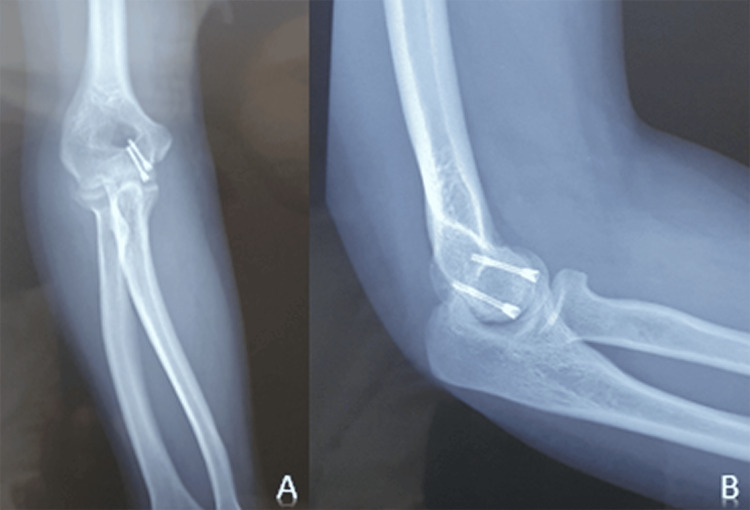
Follow-up standard radiograph in anteroposterior (A) and lateral (B) views showing fracture consolidation at two months
